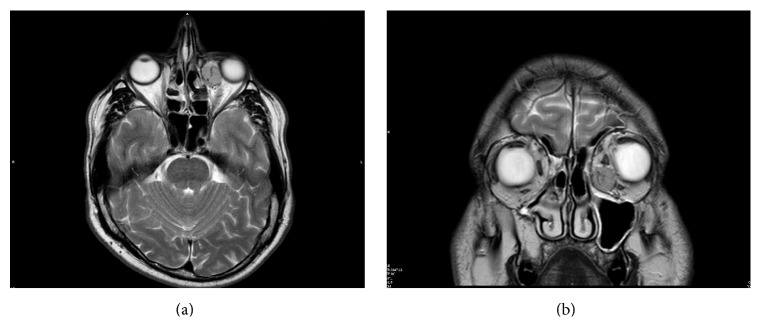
RMI: (a) Coronal T2 MRI scan shows an oval, encapsulated, vascularized, inferomedial, and extraconal mass in the left orbit. The mass displaces the eyeball and the inferior rectus muscle laterally and the medial rectus muscle cranially. (b) Axial T2 MRI scan shows the mass located in the anterior part of the left extraconal medial orbit.
